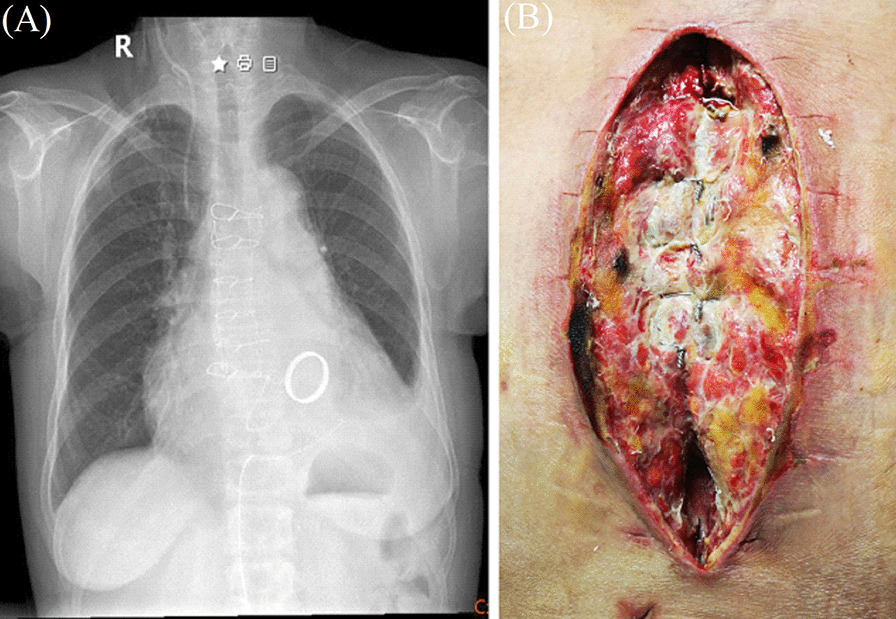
A Chest X-ray images of Robiseck sternum closure techniques. B Soft tissue ischemia after robiseck closure
